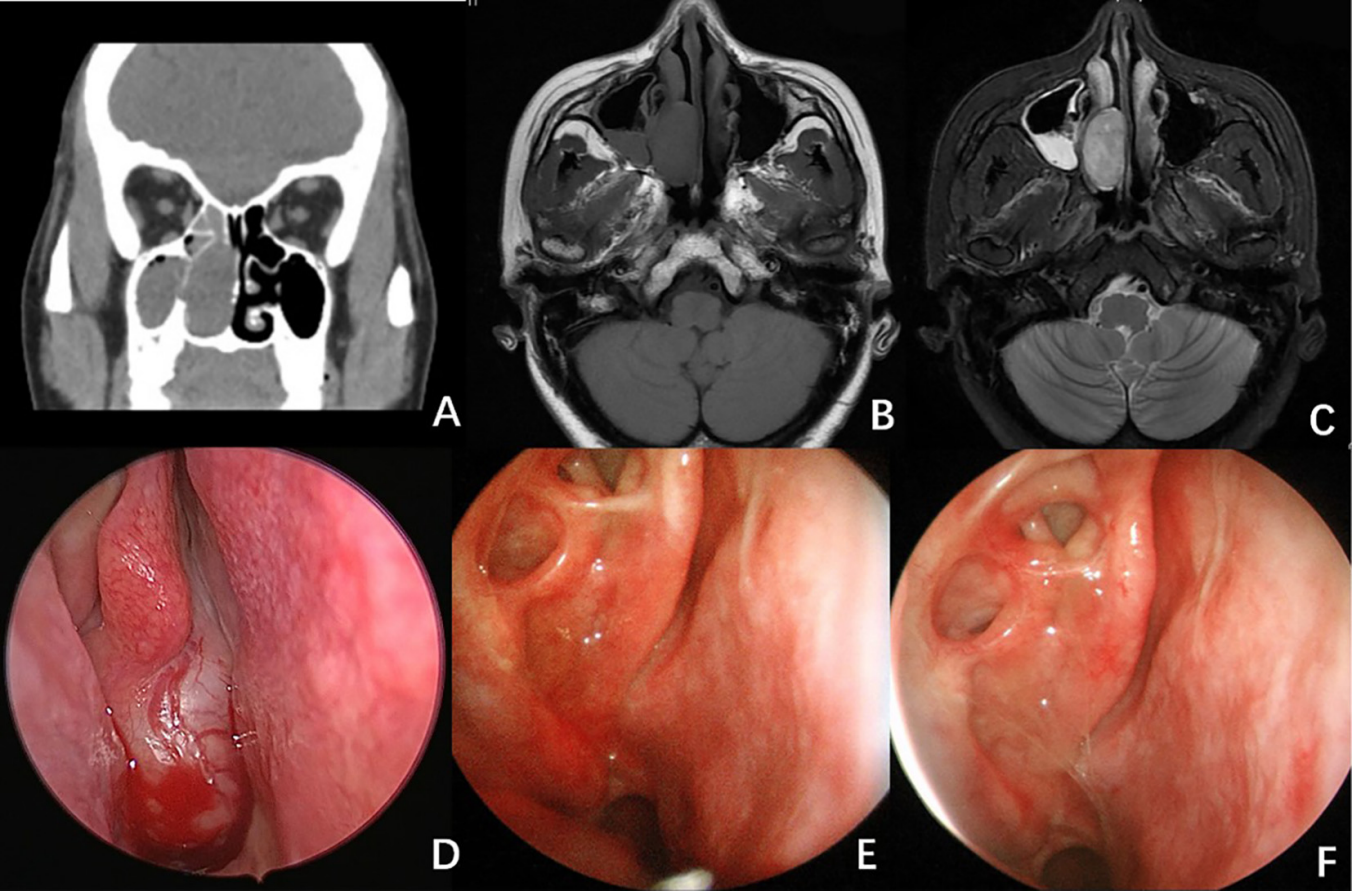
Radiological And Endoscopy Images of Case 1. (A) CT scan of the paranasal sinuses showed a lesion in the right nasal passage, measuring approximately 32×18 mm, with well-defined borders. (B, C) MRI scan showed an isointense T1 (B) and hyperintense T2 (C) signal mass in the right nasal cavity. (D) Endoscopy image revealed a neoplasm at the posterior end of the right nasal cavity, with purulent secretions on its surface. (E) Endoscopic image 5 months after surgery. (F) Endoscopic image 9 months after surgery. No signs of recurrence were observed.
